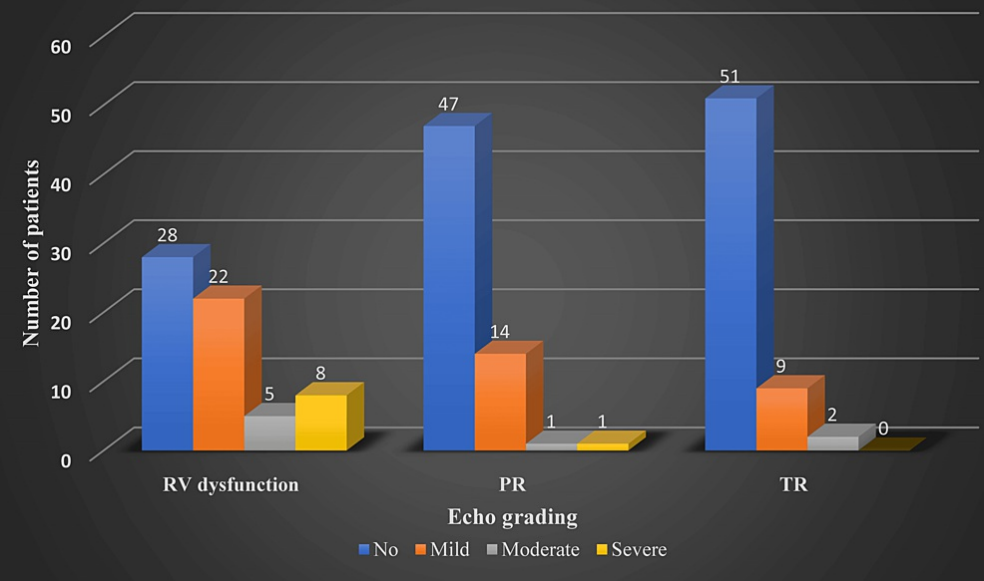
Immediate postoperative echocardiography reported complications. RV = right ventricular; PR = pulmonary valve regurgitation; TR = tricuspid valve regurgitation
